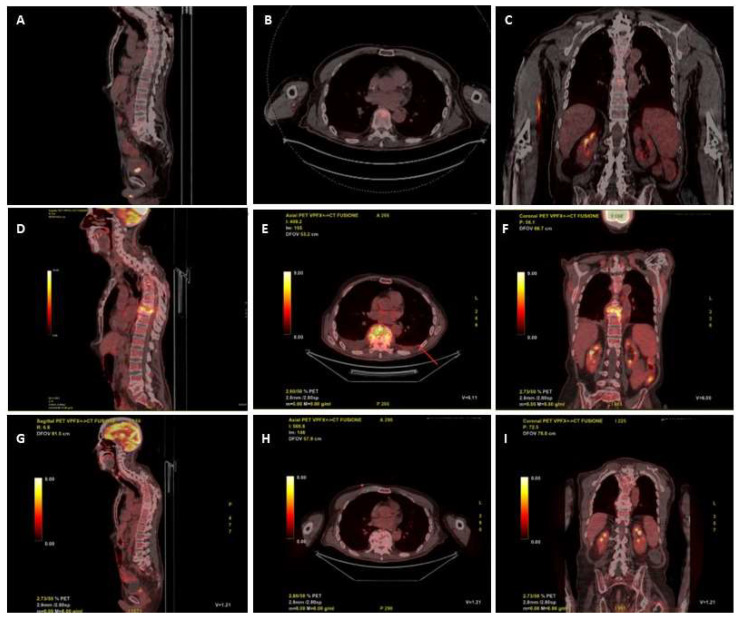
Digital PET/CT scan reconstruction (Discover GE) after administration of 250 mCi of 18-FDG: sagittal (A), axial (B) and coronal (C) planes at diagnosis of spondylodiscitis; sagittal (D), axial (E) and coronal (F) planes after 11 weeks of rezafungin: sagittal (G), axial (H) and coronal (I) planes after 26 weeks of rezafungin.
